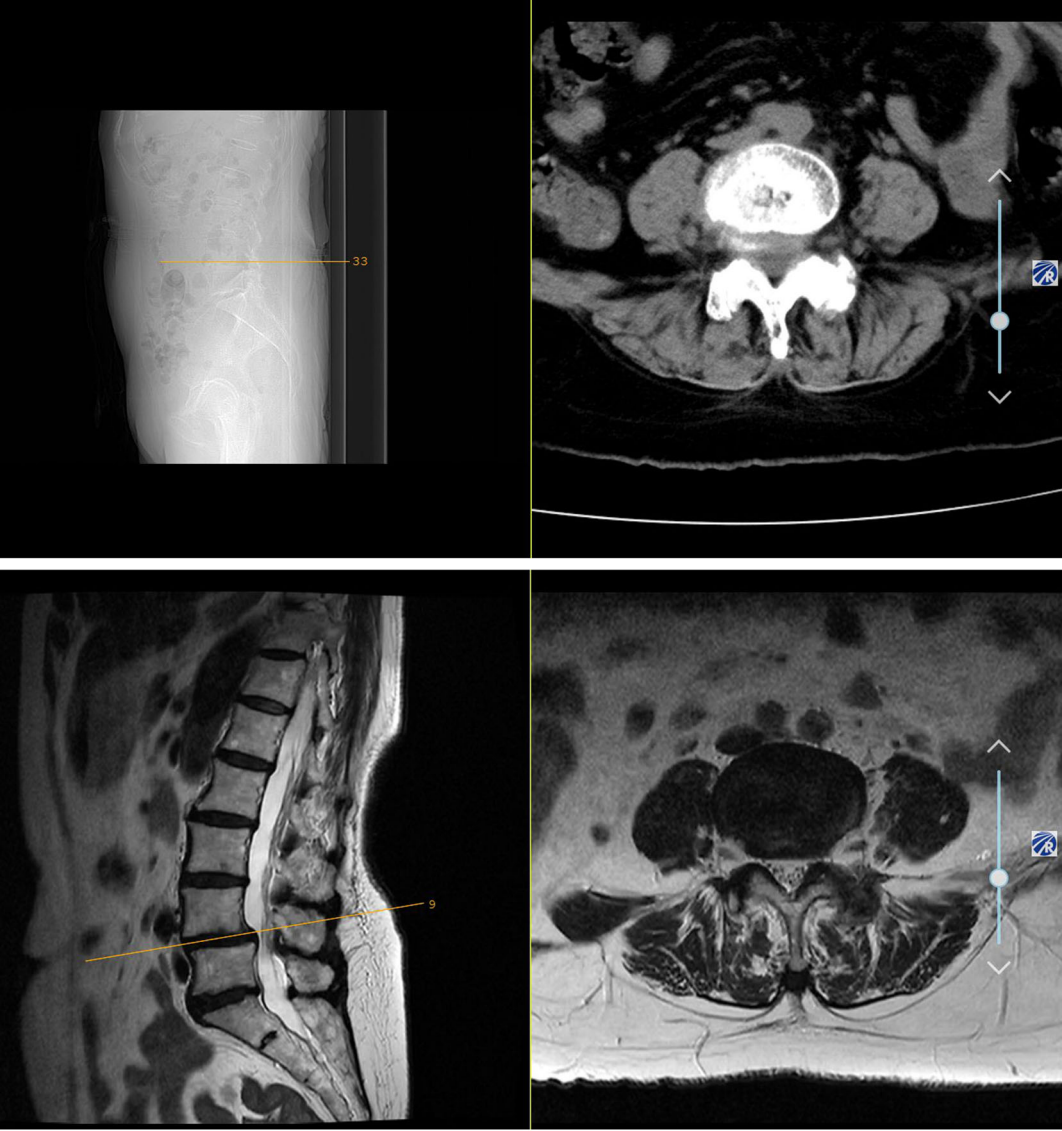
Preoperative lumbar CT scan and MRI. I degree spondyloisthesis of L_4_ and spinal canal stenosis at the L_4–5_ level.
